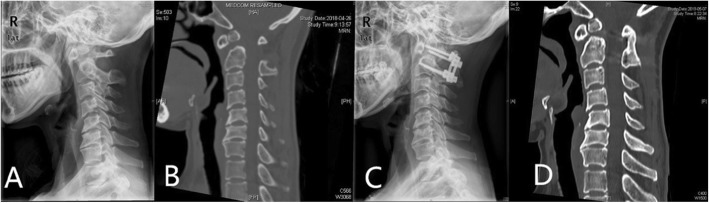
A 48-year old female suffered from progressive numbness and weakness in the left upper limb for 1 years. Sagittal X-ray (a) and sagittal reconstructed CT images of the cervical spine (b) showed os odontoideum and subluxation at the atlanto-axial joint. Posterior atlantoaxial reduction, fixation and bone graft fusion with intraoperative skull traction under general anesthesia were performed. Satisfactory reduction and fixation were achieved after surgery (c). Sagittal reconstructed CT images of the cervical spine (d) at 1-year follow-up confirmed good fusion at the atlanto-axial joint
